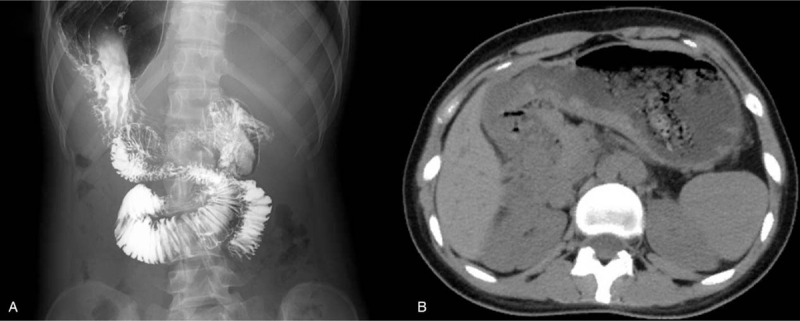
A, B Barium examination and CT scan revealed that the pyloric channel and duodenal bulb were absent. CT = computed tomography.
